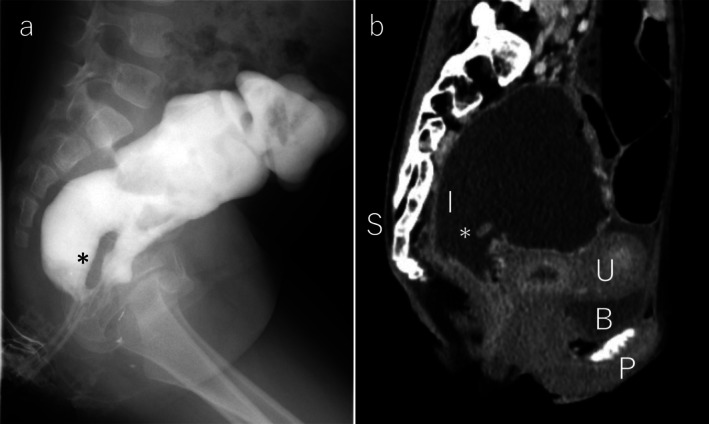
Septal formation after the Duhamel procedure (a) Contrast enema of Case 1. (b) Sagittal enhanced computed tomography of Case 2. *: Septal formation, B, bladder; P, pubis; R, rectum; S, sacrum; U, uterus.
